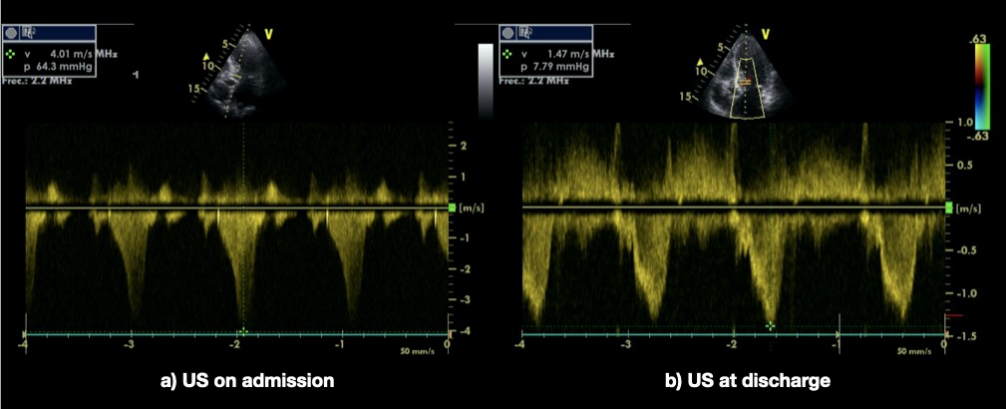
Dynamic systolic gradient in the exit tract of the left ventricle. At admission and 24 hours later. A) When it was studied using pulsed-doppler the spectral analysis had the characteristic keel shape of thesubvalvular dynamic stenosis. The gradient peak was 63 millimeters of mercury (mmHg). B) In the control echocardiography 24 hours later, there was a substantial decrease of the dynamic systolic gradient of the exit tract of the left ventricle, this was attributed to the beta-blocker treatment.
